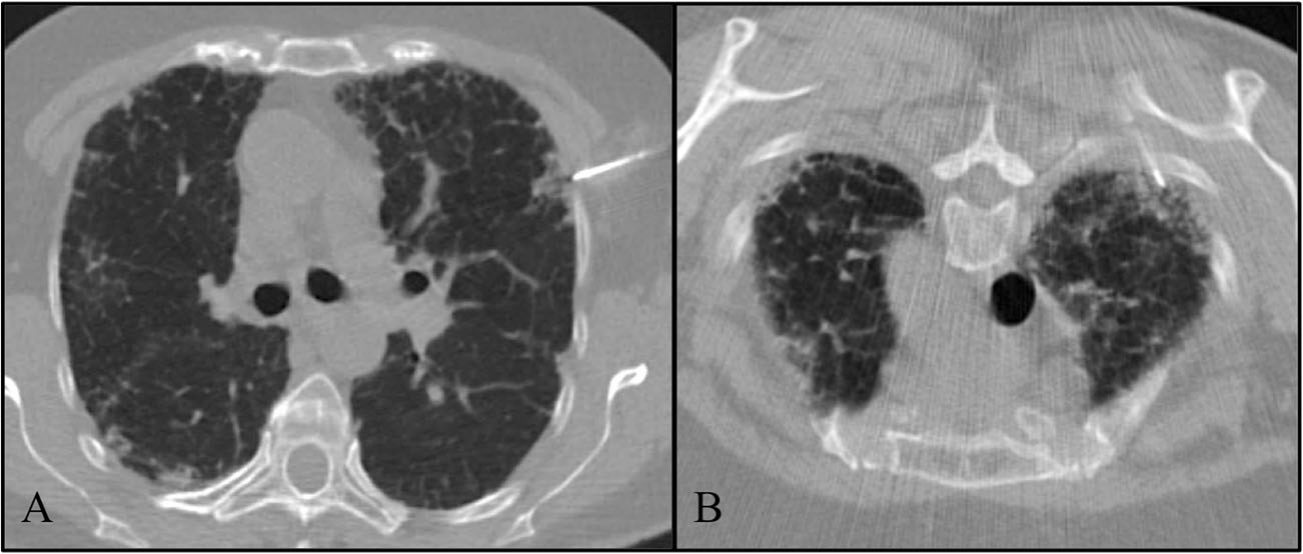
Ancillary image of a CT-guided transthoracic core lung biopsy. TTB at the upper left lobe in patient 1 (a) and TTB in patient 3 at the upper right lobe (b)
